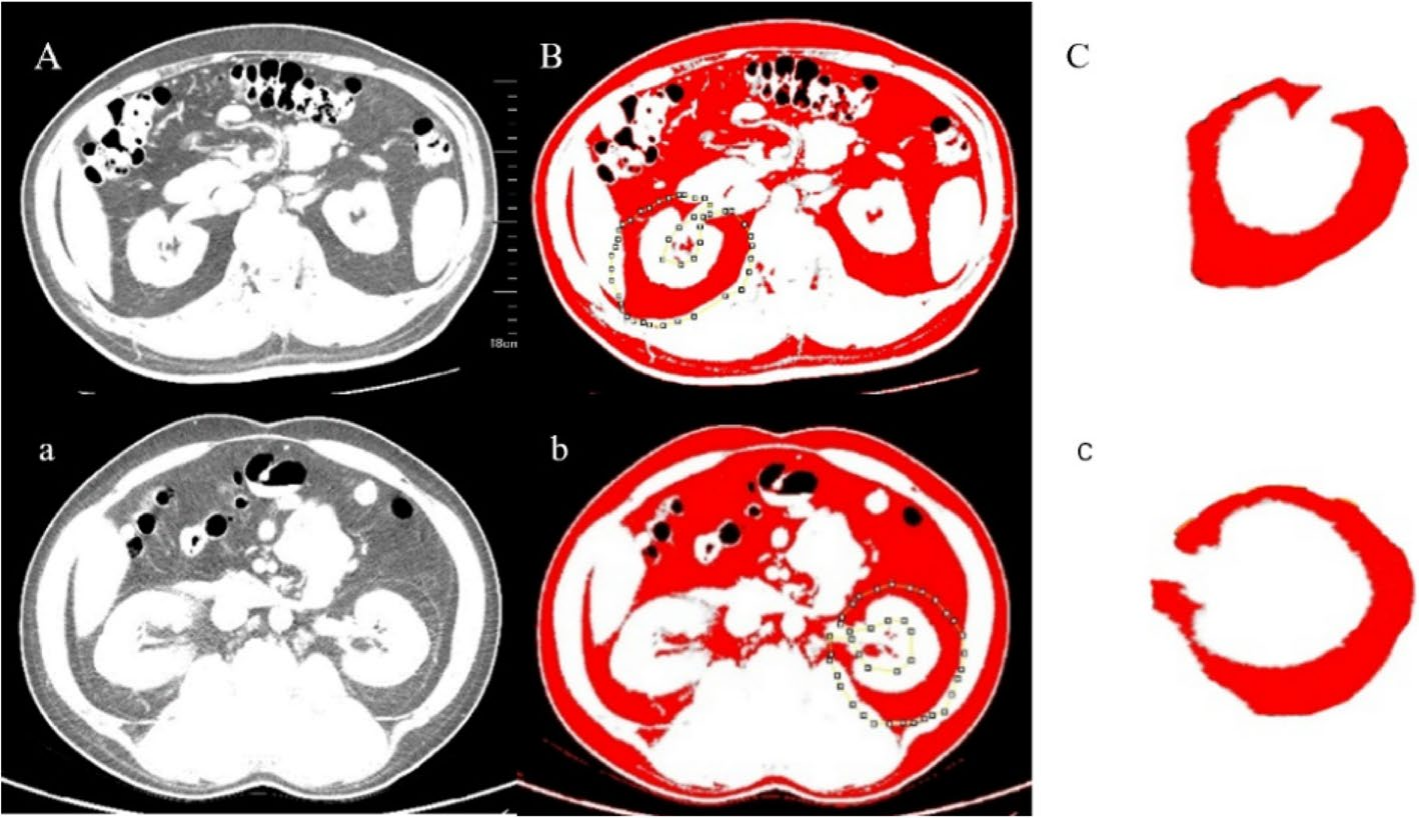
(A,a) CT images of the renal vein level; (B,b) outline the range of the perirenal fat on both sides; C: right perirenal fat area; c: left perirenal fat area
